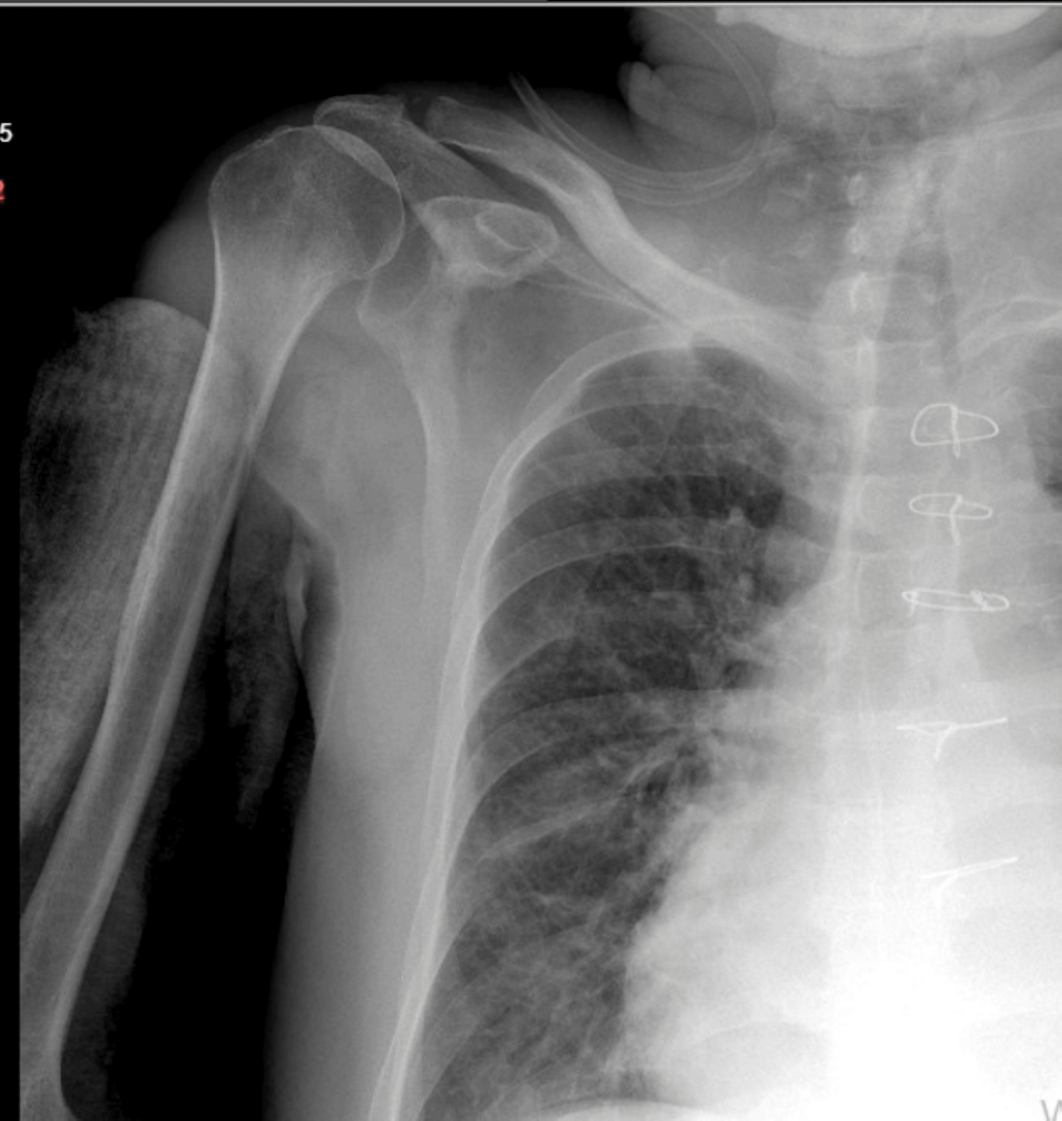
Postoperative X-ray images of the patient's right shoulder.
